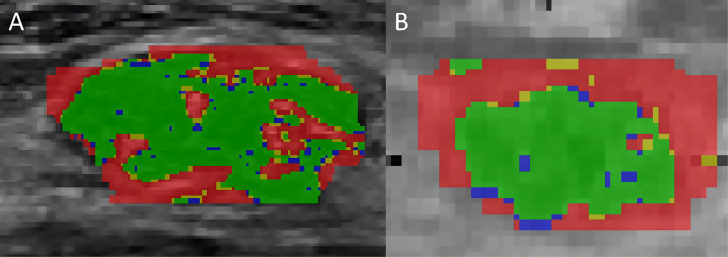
Representative visual examples of habitat subregion overlays on ultrasound images. (A) Habitat subregion overlay generated from conventional two-dimensional gray-scale ultrasound (2D-US) images. (B) Habitat subregion overlay generated from automated breast volume scanning (ABVS) images.Colors are displayed for visualization purposes only.
